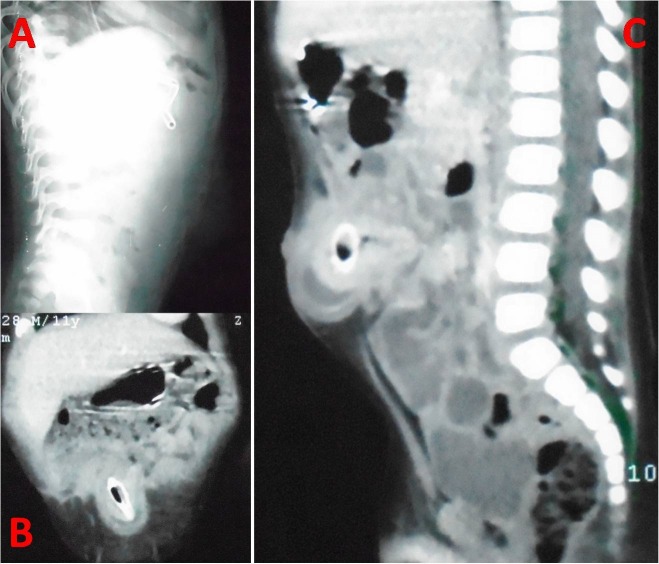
Figure 1:Preoperative radiograph showing a soft tissue shadow suggesting umbilical hernia (lateral view, A); Abdominal computed tomography scan images showing a foreign body as content of the umbilical hernia (B, C).
